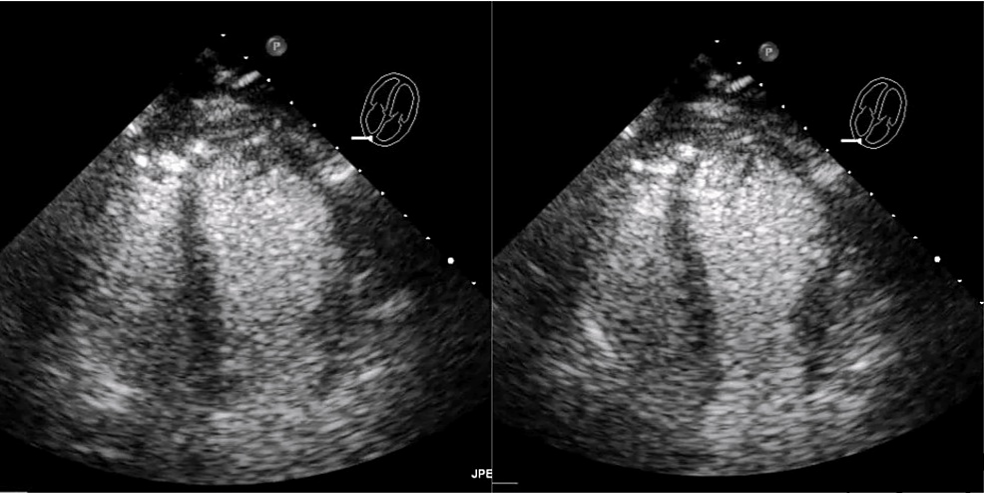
Transthoracic echocardiography in an apical four-chamber view shows contrast enhancement in systole and diastole, showing visually reduced EF and an intact interventricular septum EF: Ejection fraction.
